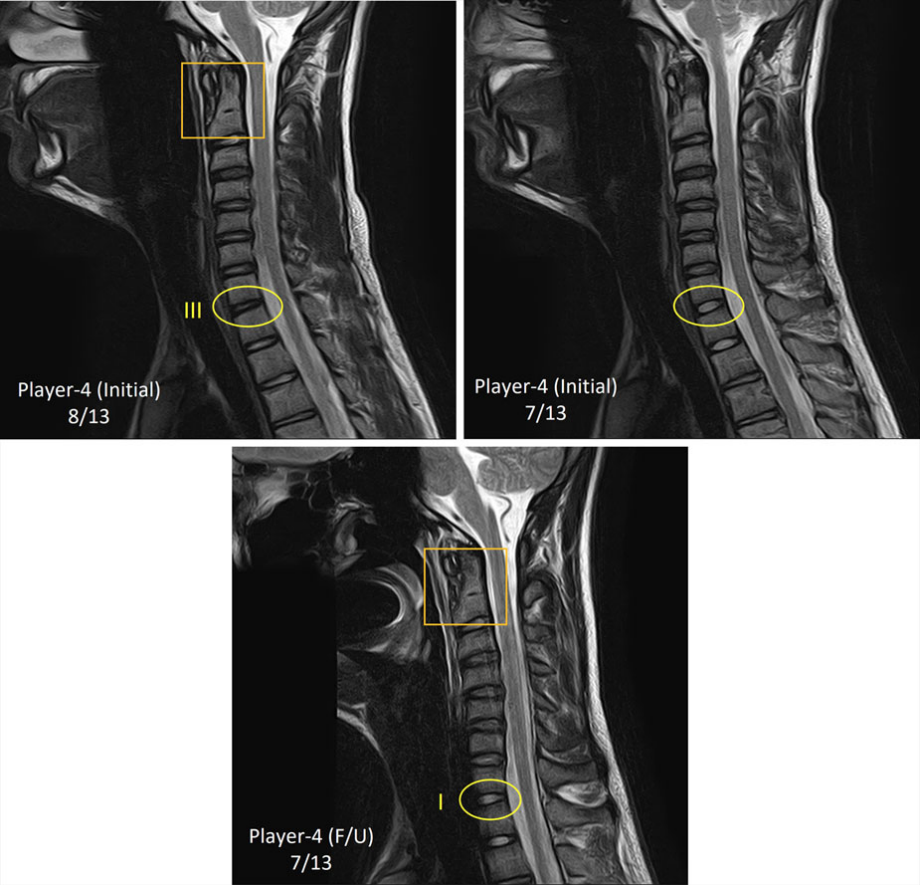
Mid-sagittal T2w image (top left) read by the readers and the image from the adjacent slice (top right) in the initial c-spine MRI and the image in the follow-up c-spine MRI (bottom) of one of the four players with repeated c-spine MRI. Selection of the sagittal slice for degenerative reading is based on visualization of the atlas (C1, orange square). In the initial scan of this subject, the adjacent slice would have been the true mid-sagittal for the cervical disc at the C7/T1 level and given a lower degenerative grade (very likely grade I) than the assigned (grade III). In the follow-up c-spine MRI, the mid-sagittal slice that was selected for the read (orange square) is the true mid-sagittal slice for the cervical disc at the C7/T1 level and yielded grade I.
